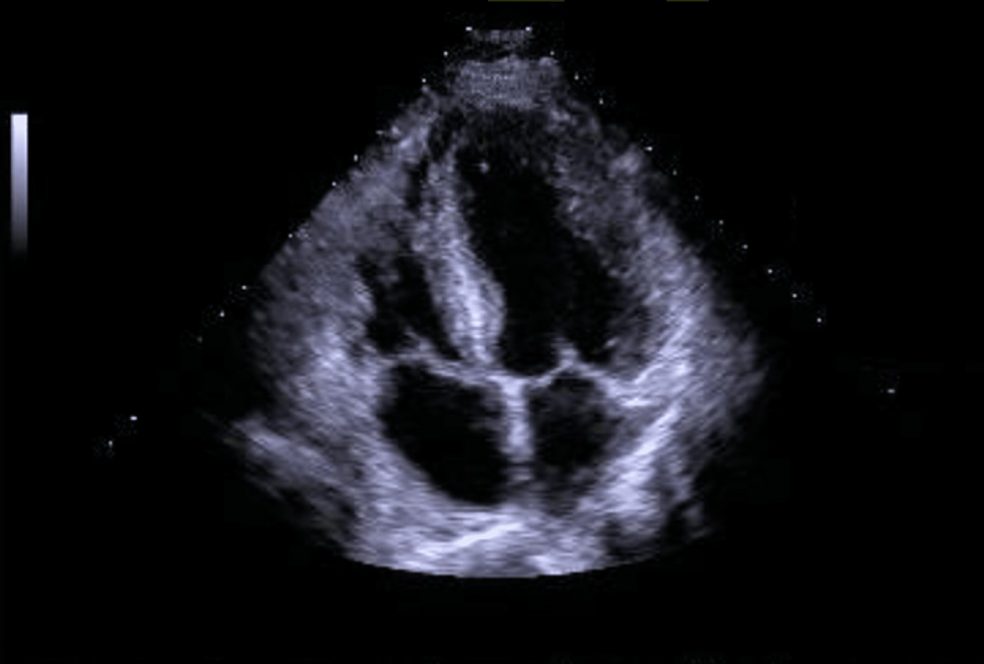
Four-chamber transthoracic echocardiogram showing mild left ventricular hypertrophy.
